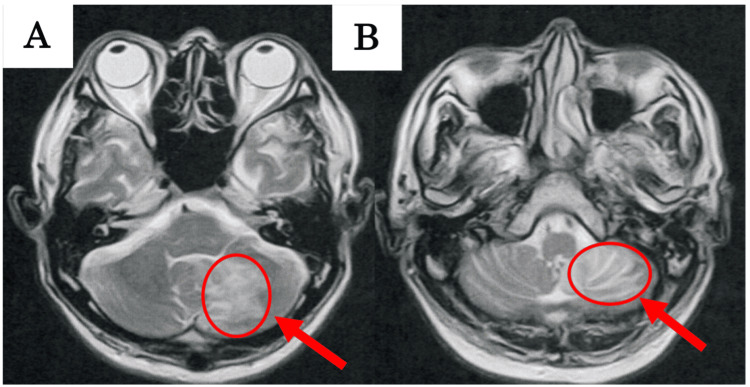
MRI at the initial assessment showing cerebellar infarction A: MRI image at the level of the middle cerebellar peduncle; B: MRI image at the level of the inferior cerebellar peduncle The arrow indicates the presence of an infarction in the flocculonodular lobe within the cerebellum.
